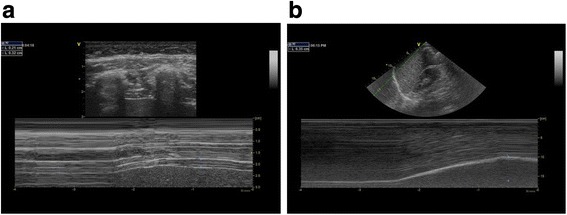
Examples of conventional assessment of diaphragm function. a Diaphragm thickening Fraction = diaphragm diameter at end-inspiration minus diaphragm diameter at end-expiration divided by diaphragm diameter at end-expiration, expressed as a percentage: [(DDinsp – DDexp)/DDexp] x 100. b Diaphragm caudal displacement with inspiration measured with a phased array transducer with the use of M-mode ultrasound in mid-axillary line in subcostal position
